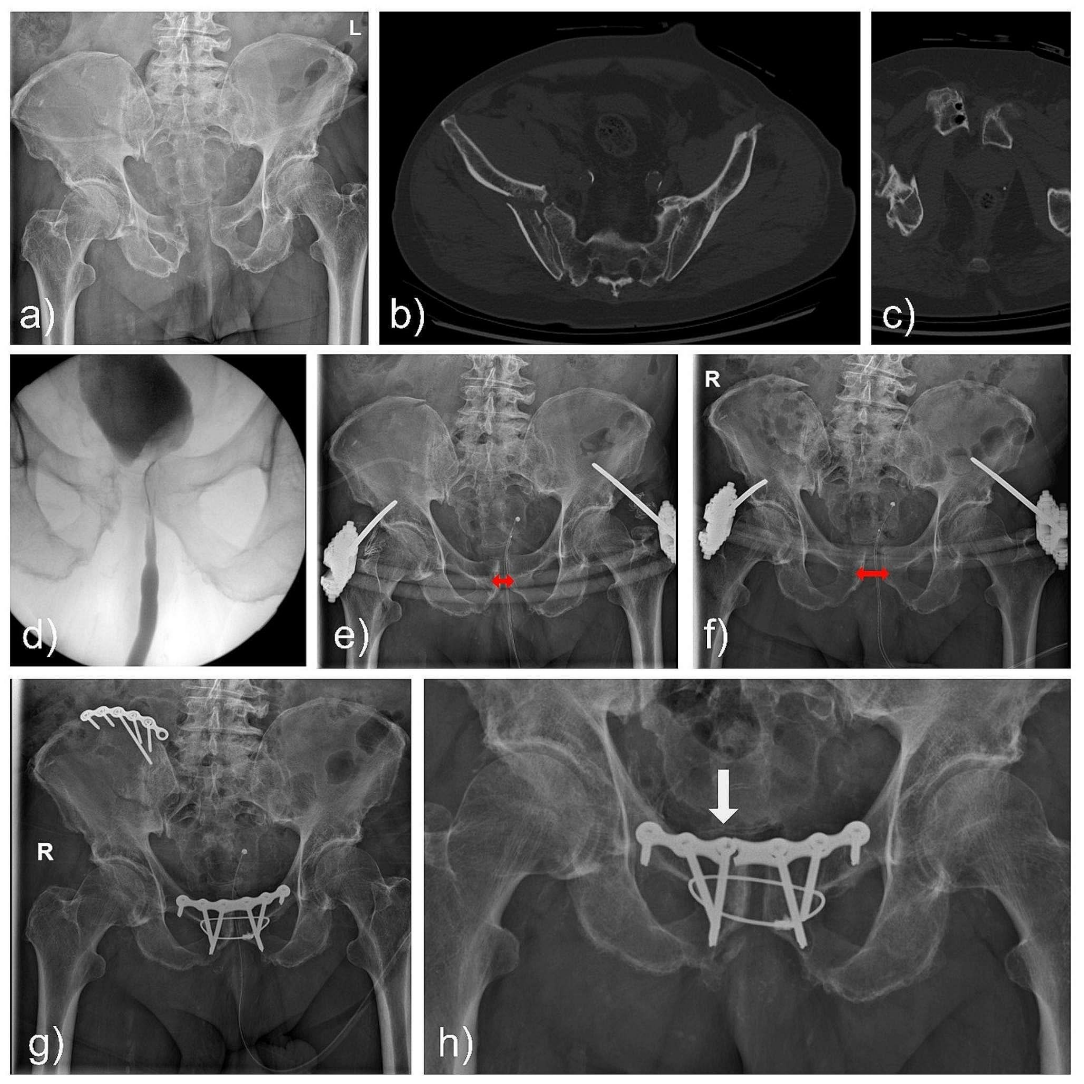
Case#4: a-c) X-ray and CT scan of a pelvic ring injury including disruption of the pubic symphysis and fracture of the ileum (AO: 61-C1.1d) in a geriatric patient. Bone cysts within the superior pubic ramus indicate already an impaired bone quality. d) Retrograde cystography prior to surgery did not reveal injury of the urinary system. e-f) An external fixator was used for emergency treatment, however, loss of reduction with widening of the pubic symphysis was observed within days (red arrows). g) Therefore, TOCF with symphyseal plating was performed. In retrospect, iliac wing plating appears to be insufficient for adequate posterior stabilization. h) Symphyseal plate breakage was observed during follow-up x-rays (white arrow). The cable remained in place, securing the reduction. This example highlights the benefit of TOCF
